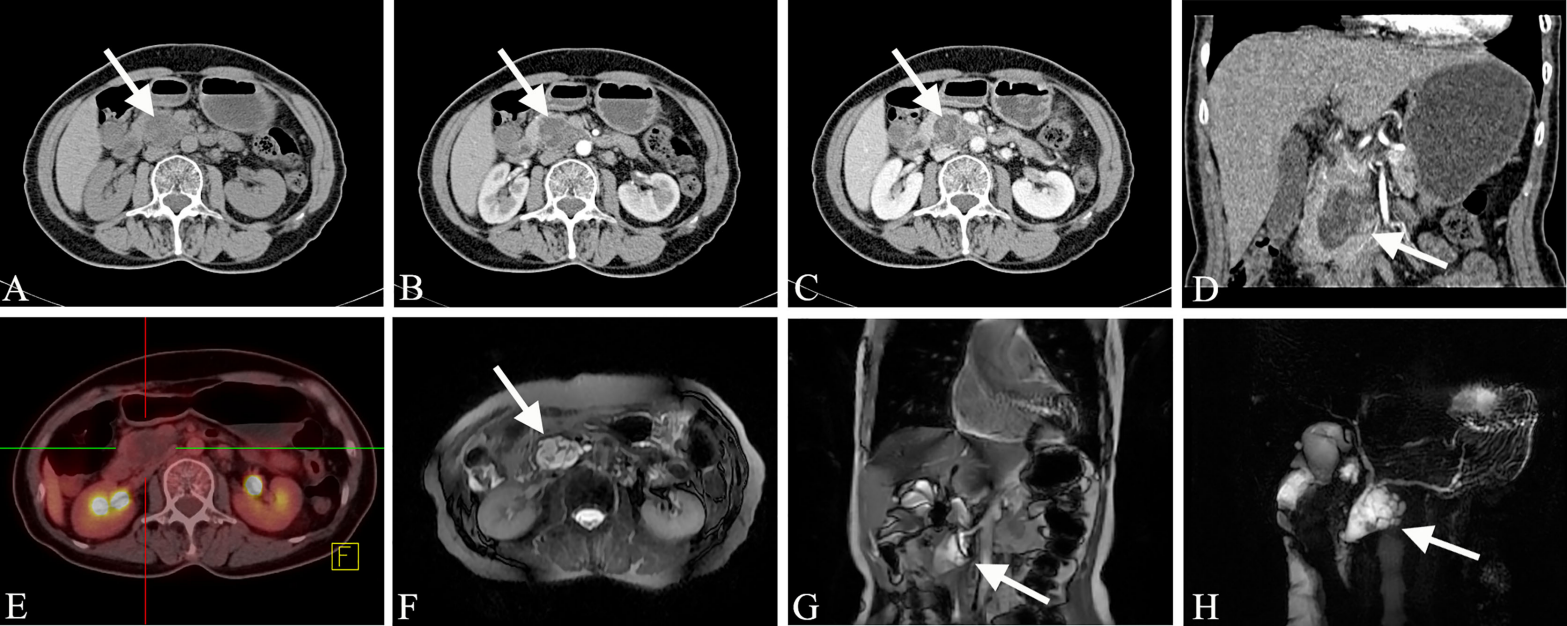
Mixed-type IPMN (Female, 56y, abdomen pain for half year). (A–C) The CT plain scan, arterial phase and venous phase at the same level; (D) Coronal image in arterial phase. (E) PET cross-sectional image; (F) T_2_-weighted cross-sectional image; (G) T_2_-weighted coronal image; (H) MRCP reconstruction map; (A) The CT plain scan showed multilocular cystic mass of pancreatic head with septation and clear boundary. The density is slightly higher than that of water. (B, C) Contrast enhanced scan showed moderate enhancement of mural nodules in the dilated MPD and branch pancreatic duct. (D) Diffuse dilatation of the MPD with enhanced mural nodules, which is the key to the diagnosis of mixed-type IPMN. (E) No obvious FDG uptake was found in the lesions by PET-CT. (F–H) Magnetic resonance imaging showed a multilocular cystic mass in the pancreatic head with multiple mural nodules, which communicated with the pancreatic duct. The white arrows in the Figures only indicate the location of the neoplasm.
